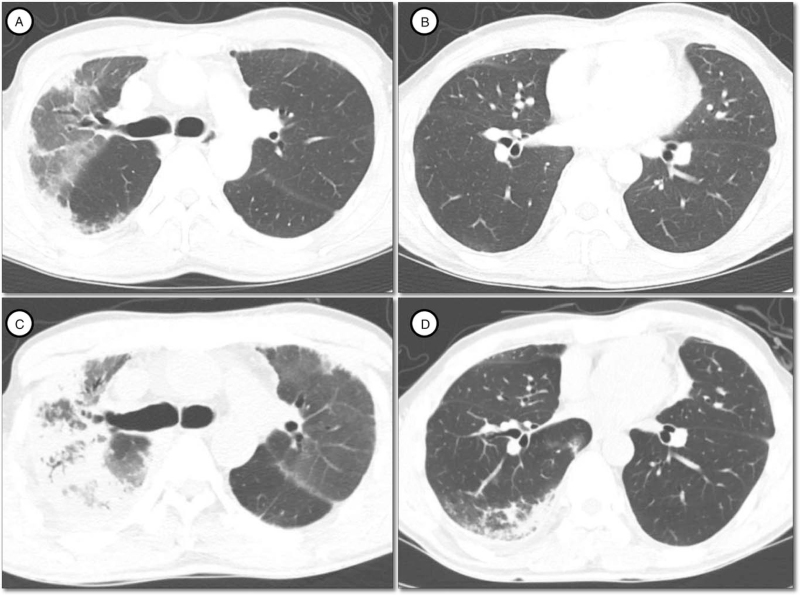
Computed tomography findings before and after the reinitiation of nilotinib. (A, B) Before the reinitiation of nilotinib, computed tomography demonstrated persistent fibrotic interstitial lung disease in the right upper lobe. (C, D) Six months after the reinitiation of nilotinib, computed tomography demonstrated aggravated subpleural consolidations with traction bronchiectasis in the right upper lobe and newly developed subpleural consolidation in the right lower lobe.
